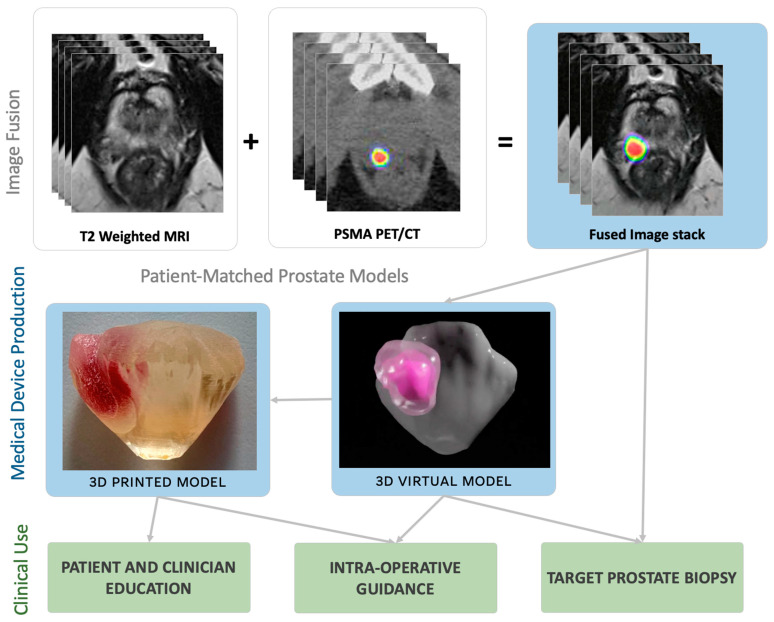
Clinical utility pathways for applying the fused PET/MRI image fusion in urological practice.
